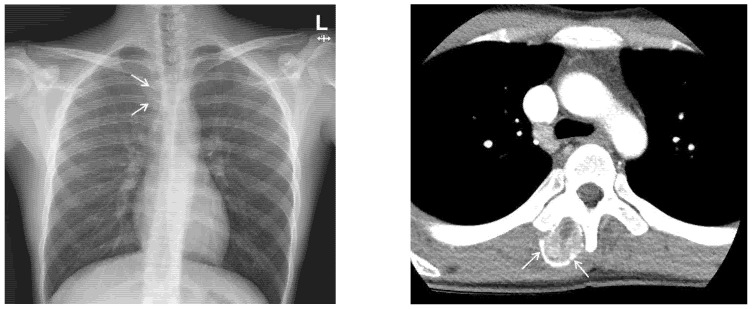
(Left) Chest X-ray demonstrates radiopaque mass (arrows) with rim calcification in the T4/5 paraspinal area. (Right) Axial CT scan shows an oval-shaped mass with peripheral rim calcification (arrows) in the right paraspinal muscle.
